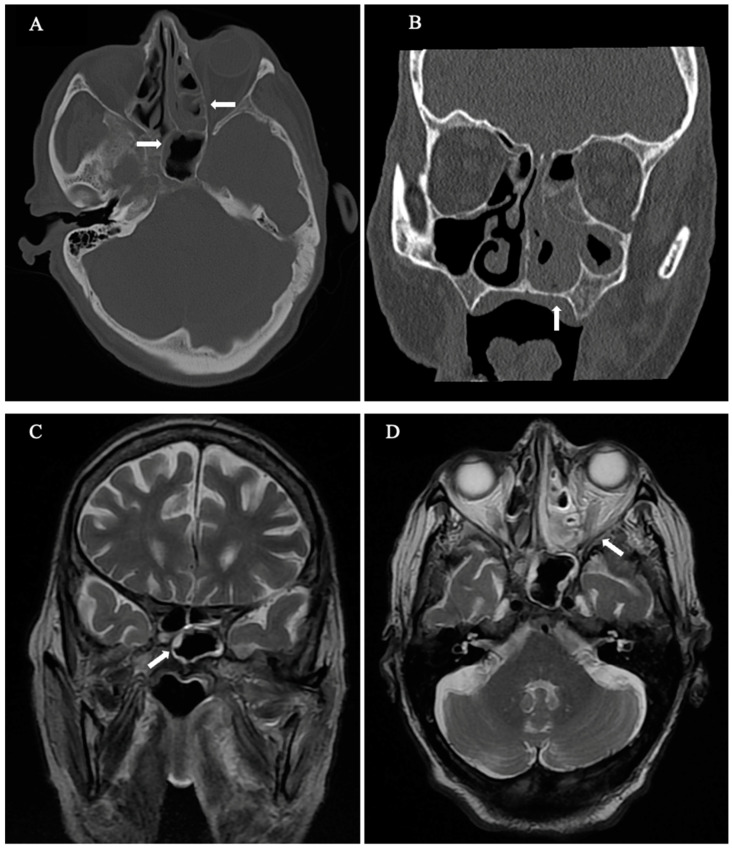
(A–D) CT and MRI scans show an inflammation of the paranasal sinuses without erosion of the petrous bone (note that bone destruction in these cases can be extensive, subtle, or even inapparent). (A) Axial CT: Moderate to severe mucoperiosteal thickening predominantly in the posterior ethmoidal cells, with subtotal cell occupancy (horizontal arrows). (B) Coronal CT: Subtotal occupation with mucoperiosteal thickening and soft tissue attenuation in ethmoidal and maxillary sinuses as well as in the left nasal cavity (vertical arrow). (C) Coronal T2 WI MRI: Mild hyperintense mucosal peripheral thickening of the sphenoid sinus (diagonal arrow). Note central hypointensity, which might be due to either air or fungal elements. (D) Axial T2 WI MRI: hyperintense peripheral soft tissue in the left paranasal sinuses with central hypointensity within the posterior ethmoidal cells, consistent with fungal elements (diagonal arrow). No fat stranding indicating invasion outside the sinus.
